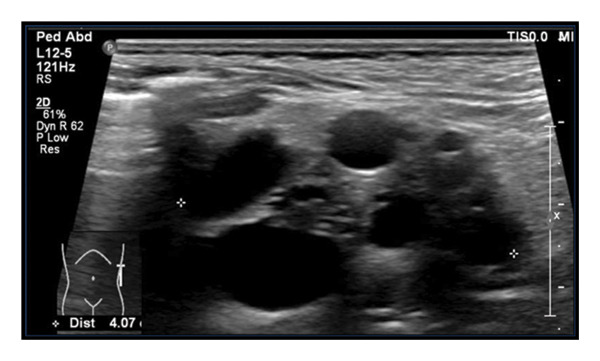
Renal Cysts. An 8‐month‐old boy with a multicystic dysplastic kidney. On ultrasound, multiple cysts are present, and no normal renal tissue is seen.
